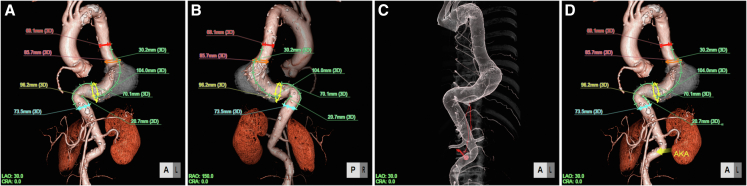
Volume-rendered images of computed tomography angiographies (CTAs) in a 70-year-old woman with aortic aneurysm. The volume-rendered images of standard CTA with thoracic endovascular aortic repair (TEVAR) planning are shown (A, B). The aneurysmal section is marked in *orange to yellow*, the proximal landing site of the TEVAR plan is *red*, and the distal site is *light blue*. The measured values represent the inner perimeter of the true lumen at each site. In the standard CTA, the Adamkiewicz artery (AKA) was not identified, and the distal landing was at zone 5, posing a potential risk of spinal cord ischemia (SCI) due to possible AKA coverage. (C) The AKA-specific CTA (AKA-CTA) is shown, revealing the AKA originating from the right L2 segmental artery (*red-colored vessel*). The TEVAR planners were informed of this L2 branching (indicated by a *yellow arrow* in panels A and B). Although the TEVAR plan remained unchanged, TEVAR planners reassessed the SCI risk as low.
